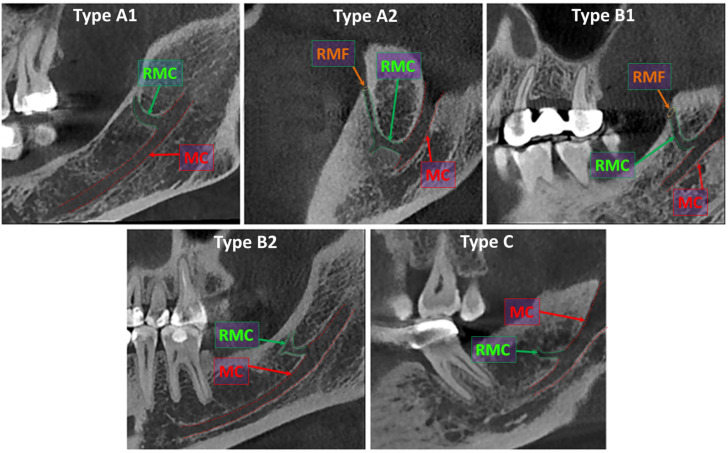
Oblique sagittal CBCT images showing the types of retromolar canals (MC: mandibular canal; RMC: retromolar canal; RMF: retromolar foramen).
